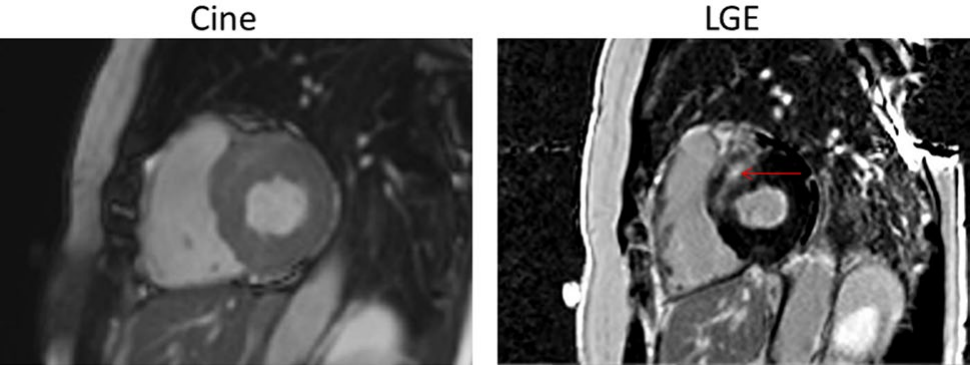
Typical example of a patient who died due to cardiovascular causes with a late gadolinium enhancement lesion on cardiac magnetic resonance imaging
